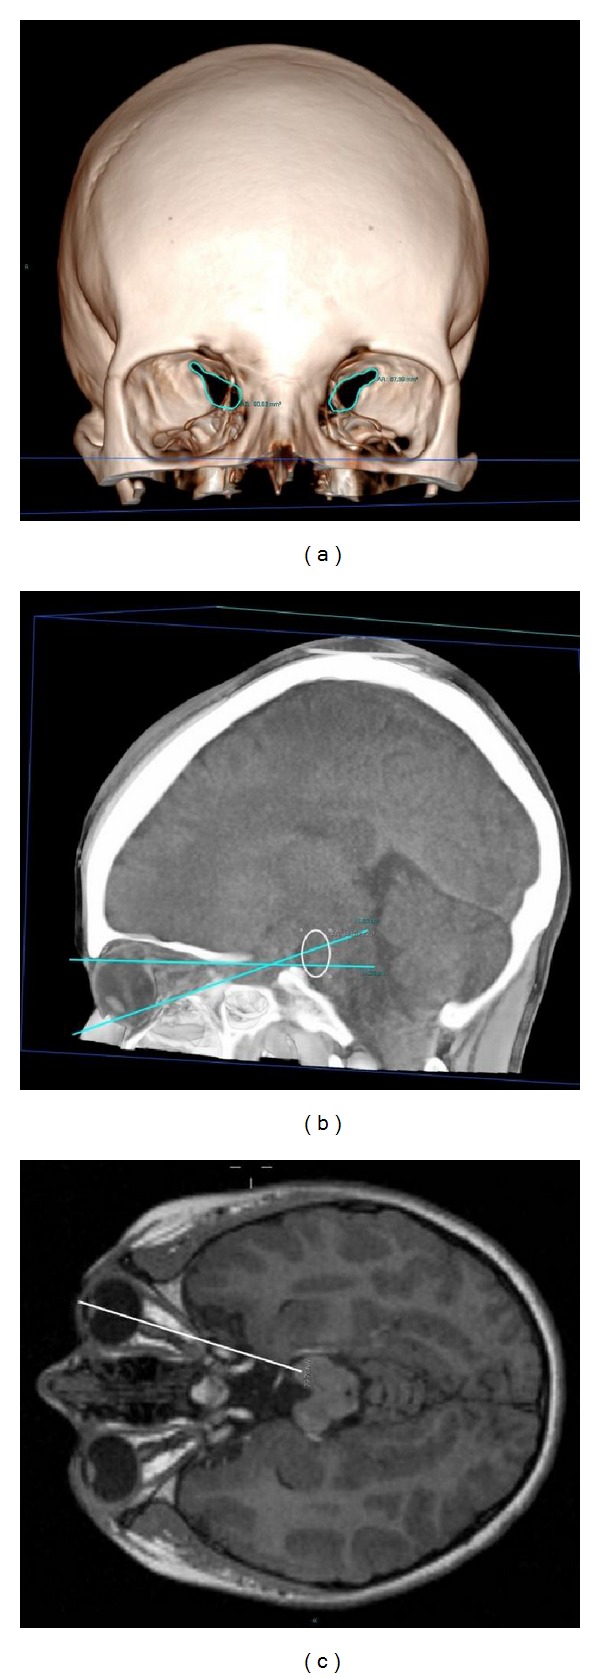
CT and MRI analysis of a human head to trace a possible pathway for light from the outside to the *substantia nigra*. (a) Human head 3D CT scan showing the large superior orbital fissure that gives access to the internal cavity of the skull. (b) Sagittal CT scan of a human head. It is evident from this prospective that the mesencephalon (indicated by the white ellipse) is behind the orbit, not hidden by the posterior clinoid process. (c) Axial MRI scan of a human head. This image shows that CSF occupies a large volume between the superior orbital fissure and the mesencephalon. Furthermore, CSF is present around the optical nerve in the intraconal space. The white line indicates the estimated distance (79.78 mm) between the cornea and the mesencephalon.
